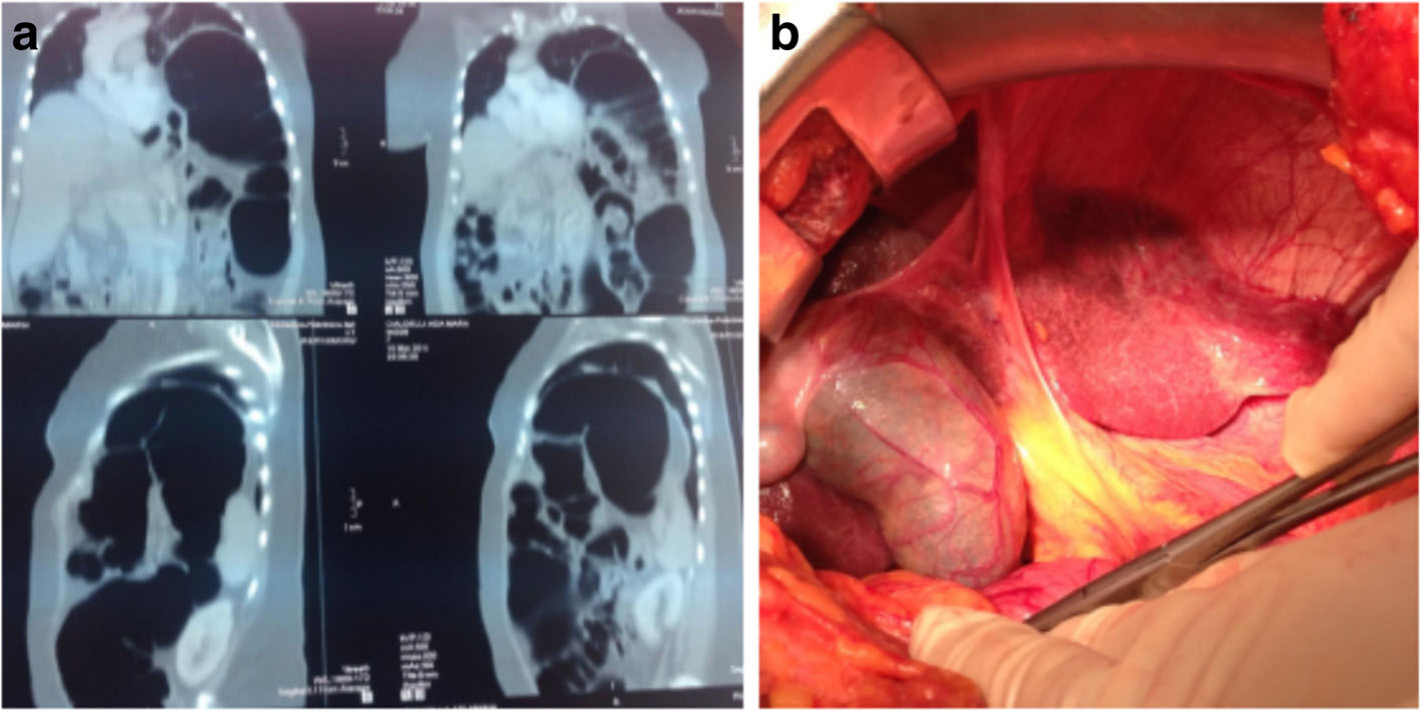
a CT scan shows collapse of the lung and the mediastinal shift towards to the *right side*. The *left* diaphragmatic hernia contained the transverse and descending colon. b Intraoperative evidence: diaphragmatic defect allows migration of viscera
